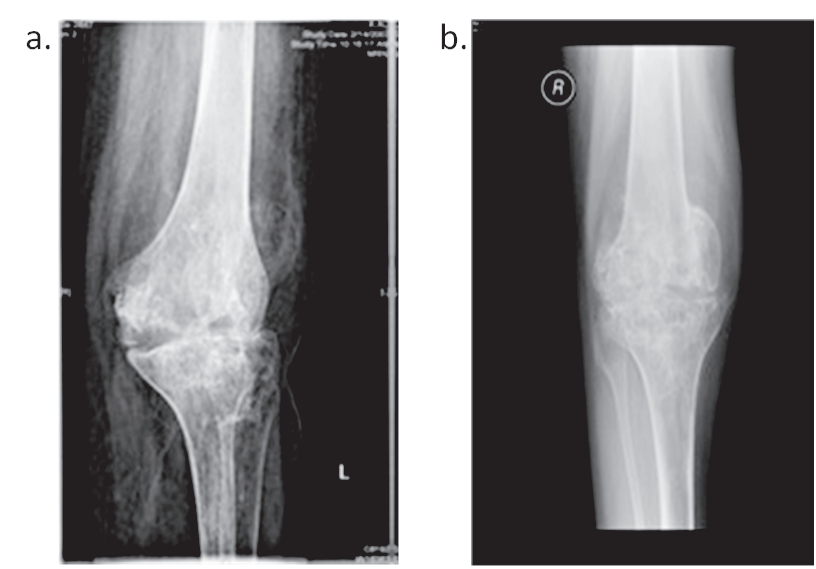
AP left & right knee x-ray shows erosion and ankylosis.
